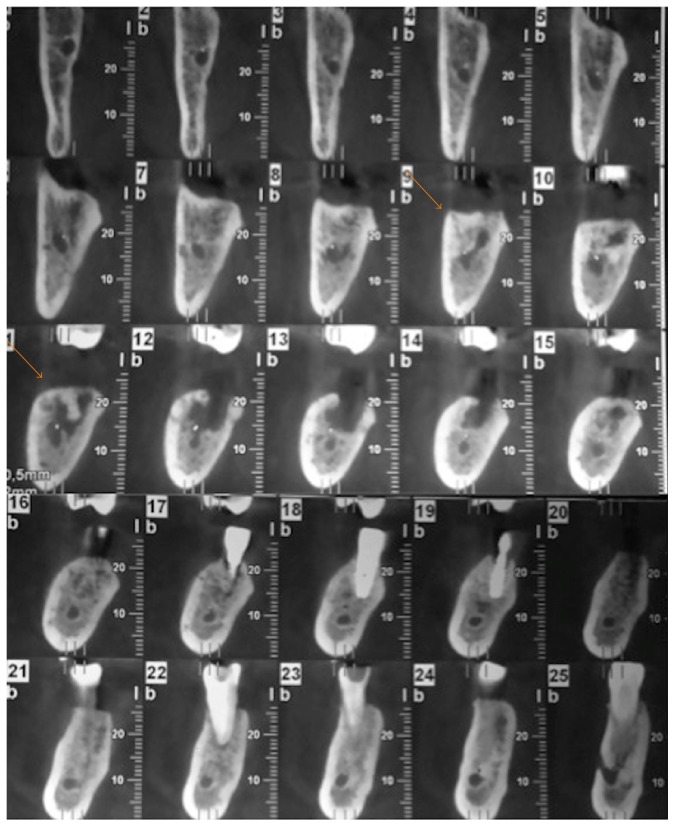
CT Dentascan scan performed on July, 2011, showing sequestrum.
